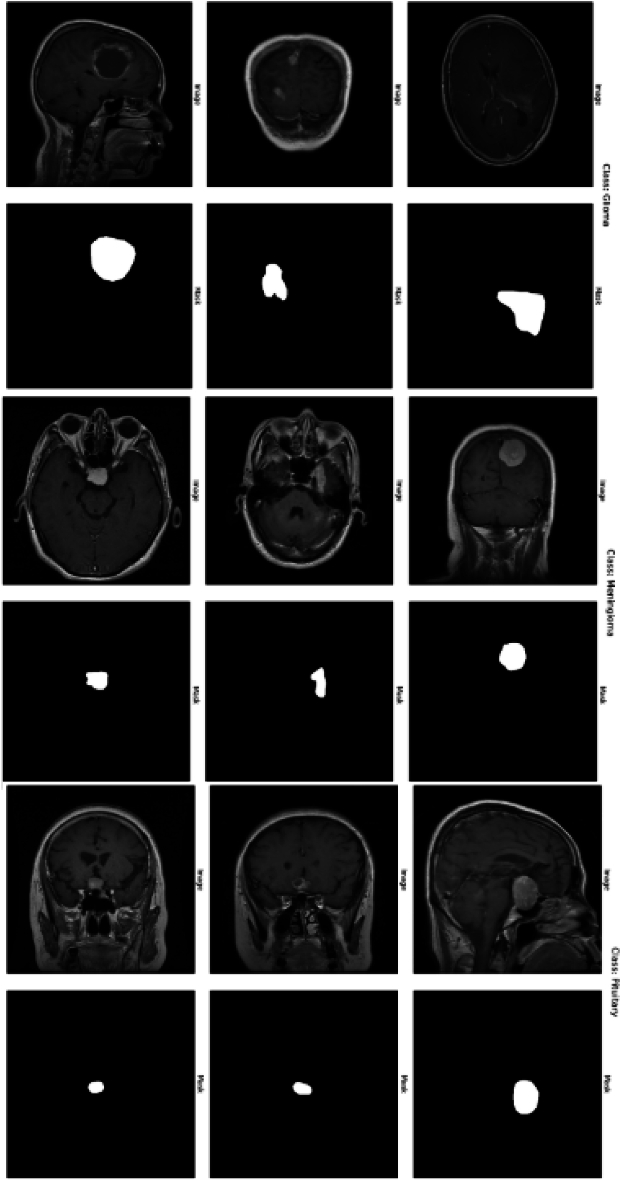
Sample MRI images and their corresponding masks in the dataset.
